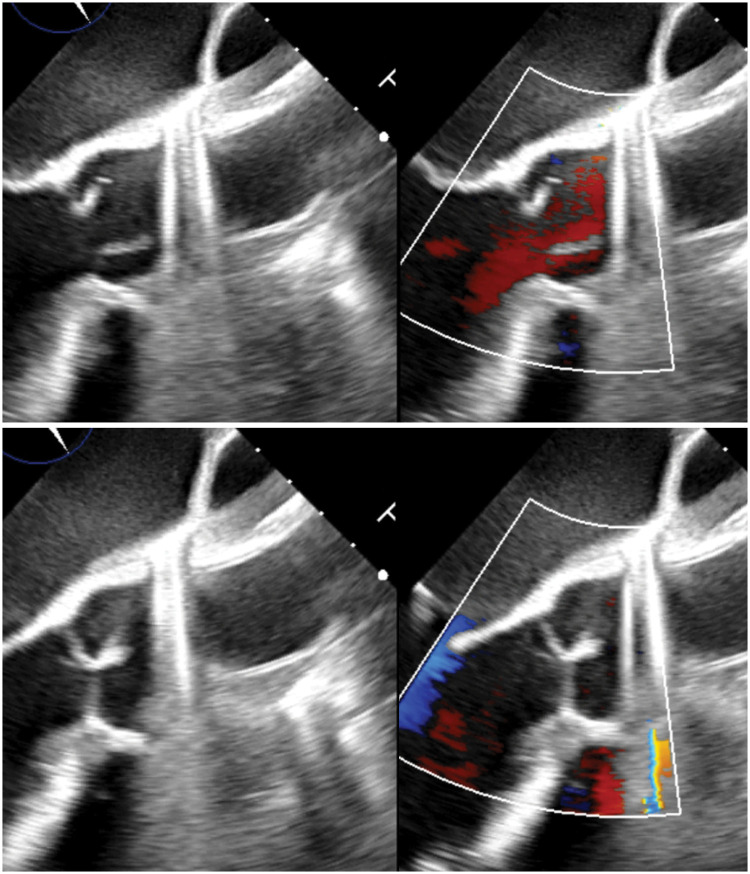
Transoesophageal Echocardiography Demonstrating Opening and Closing of the Reconstructed Bicuspid Aortic Valve by Tricuspidation
